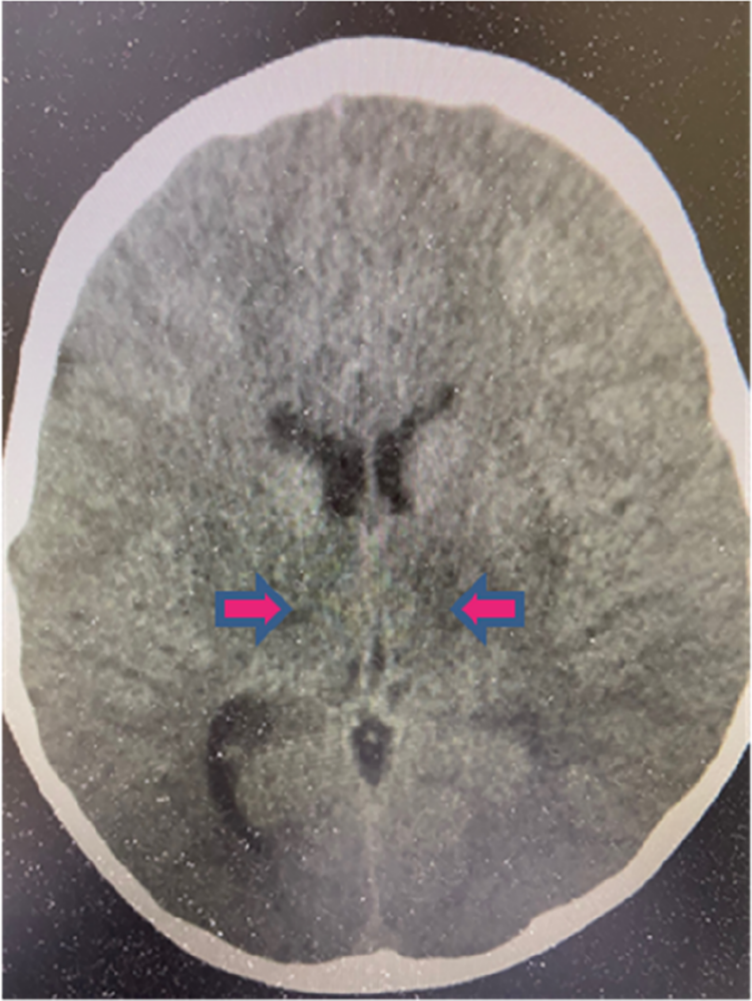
Brain CT scan of the ANE patient. The CT scan revealed hypodense edema involving bilateral thalami, dorsal pons, and midbrain.
